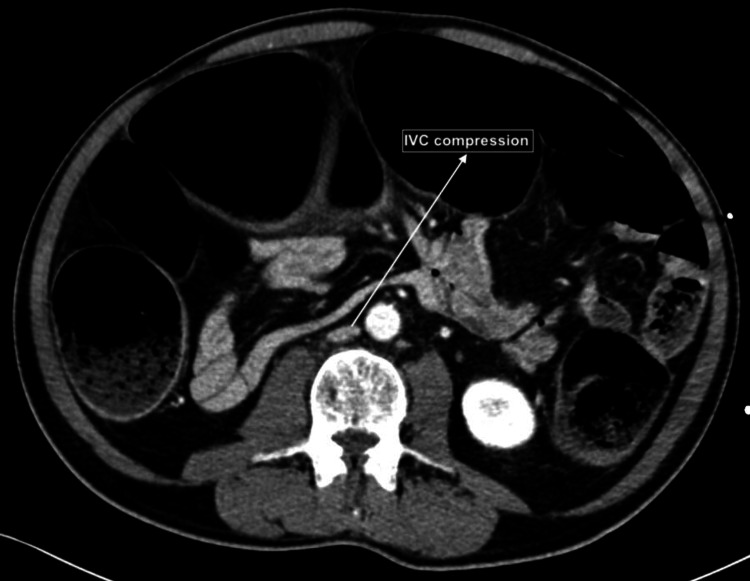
CT image of the abdomen in an axial cut showing compressed inferior vena cava (IVC)
